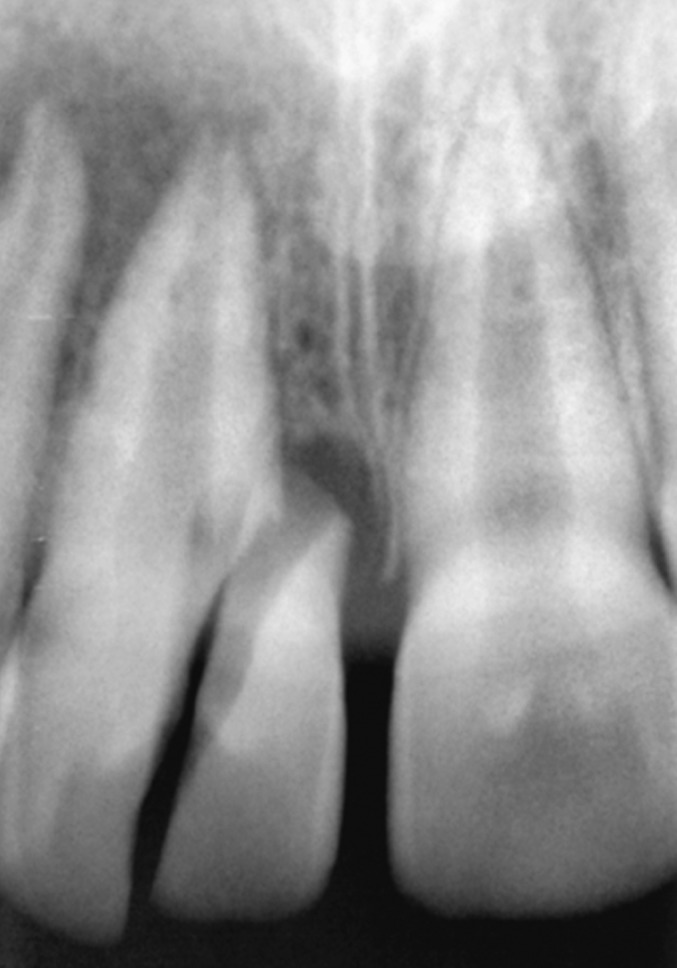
Preoperative radiograph of the fractured tooth
